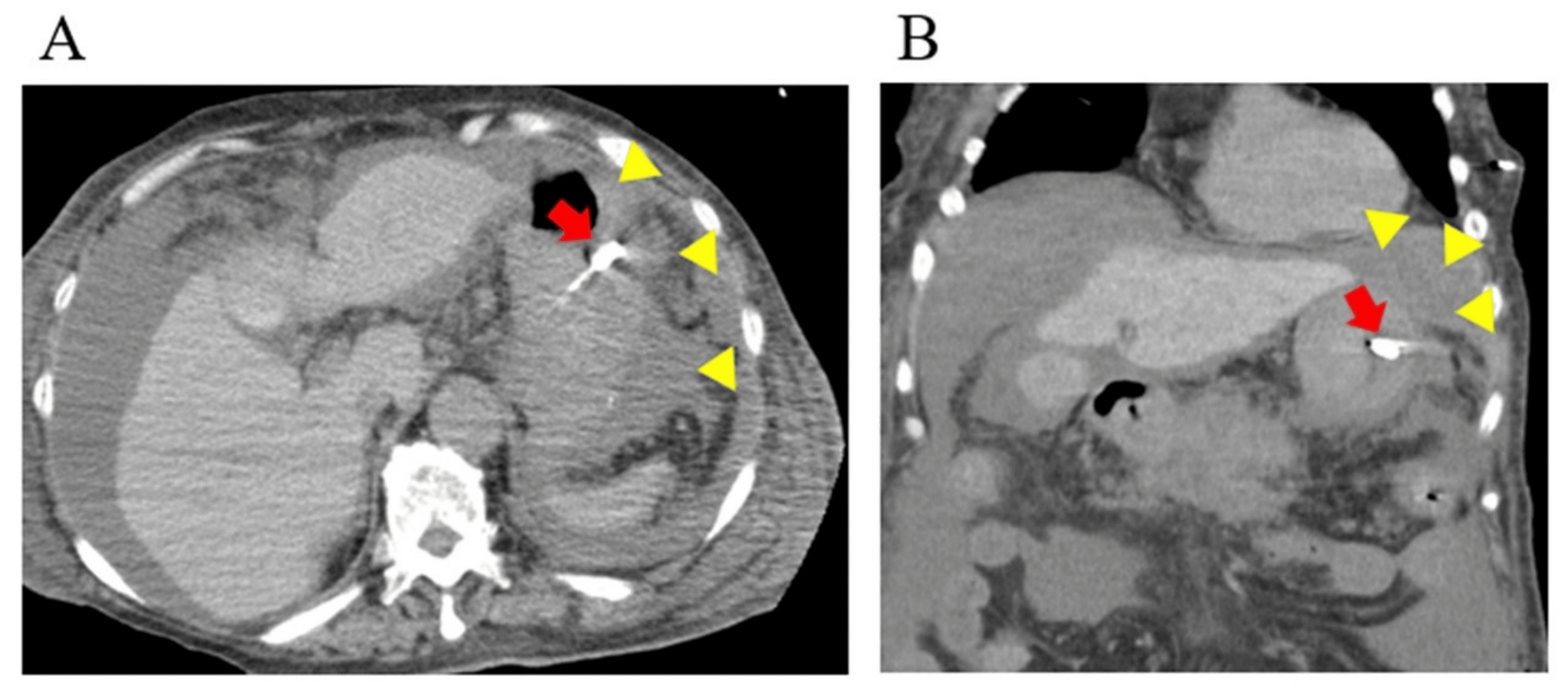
CT image on the day after the NGT placement (case 9) The image shows the NGT tip in the stomach (arrow) and massive ascites (triangle). (A) shows the axial scan, and (B) shows the coronal scan. CT, computed tomography; NGT, nasogastric tube
